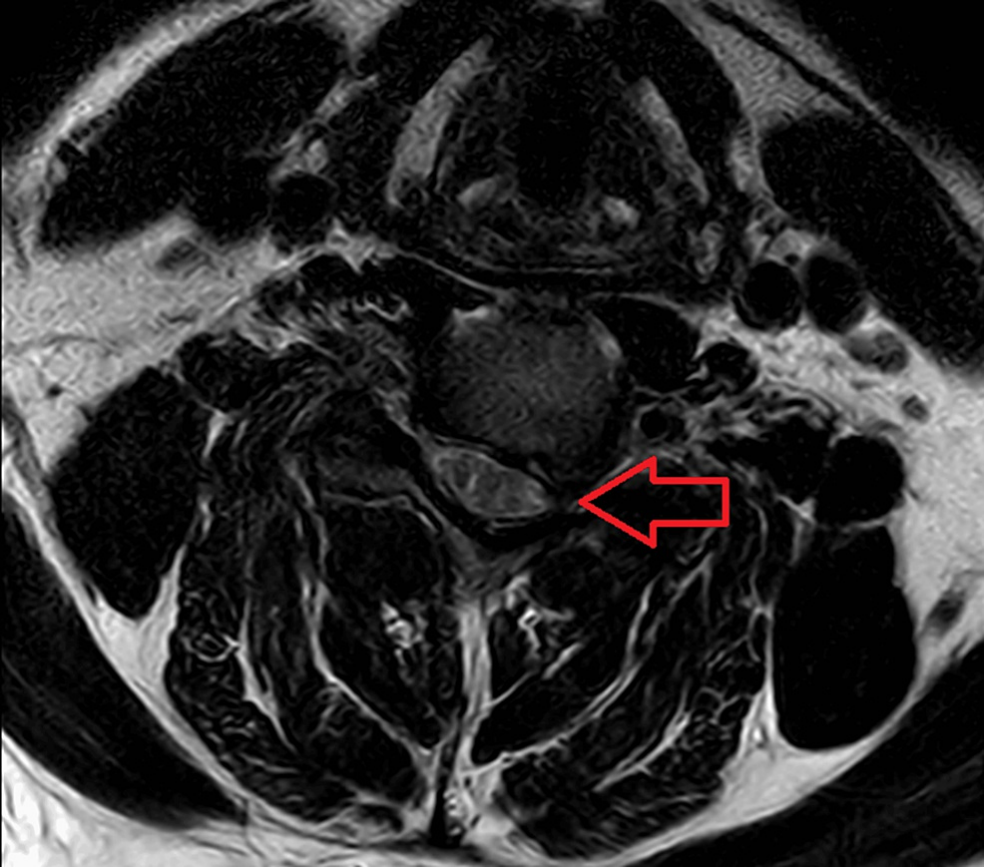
MRI (axial section) at the level of C5-C6 showing narrowing of the neural foramen causing compression of exiting and traversing nerve roots (arrow) MRI: magnetic resonance imaging
